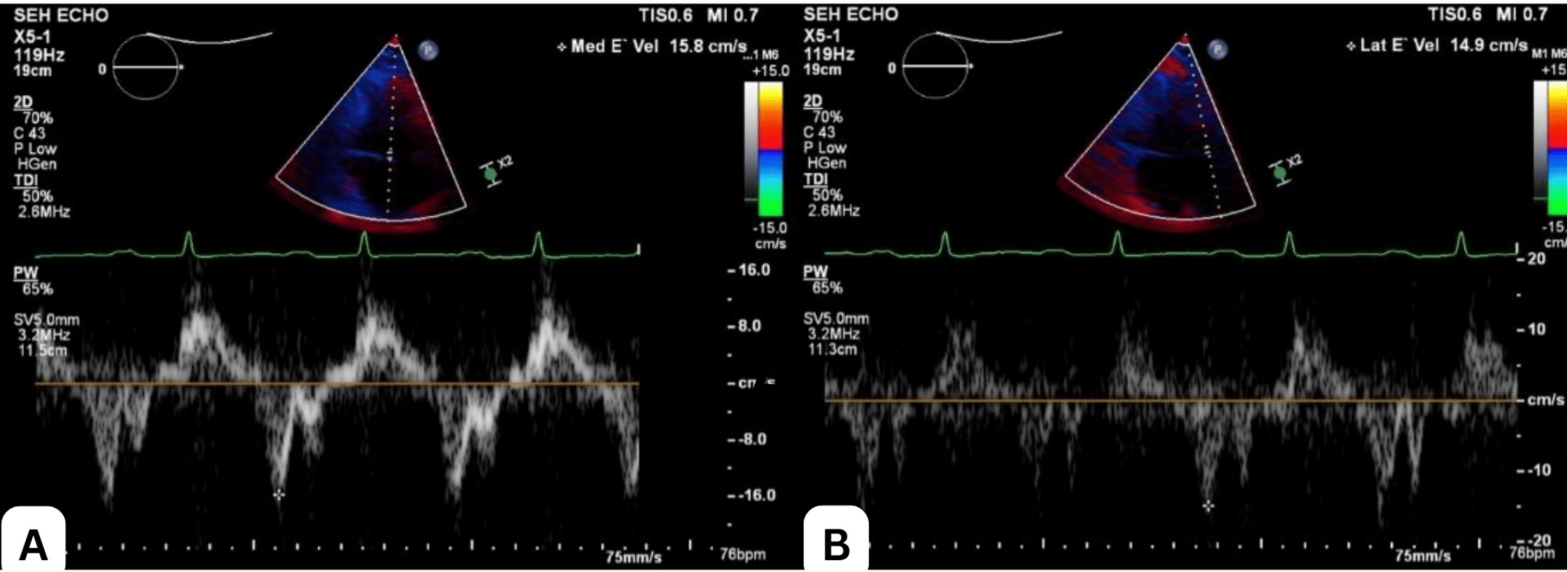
Transthoracic echocardiography (TTE) shows mitral ‘annulus reversus,’ as evidenced by mitral velocity e’ velocity >2% and higher than lateral e’ velocity, which was missed during initial TTE evaluation, the reason being preserved lateral e’ velocity. Figure A shows medial e' velocity of 15.8 cm/s, and Figure B shows lateral e' velocity of 14.9 cm/s.
